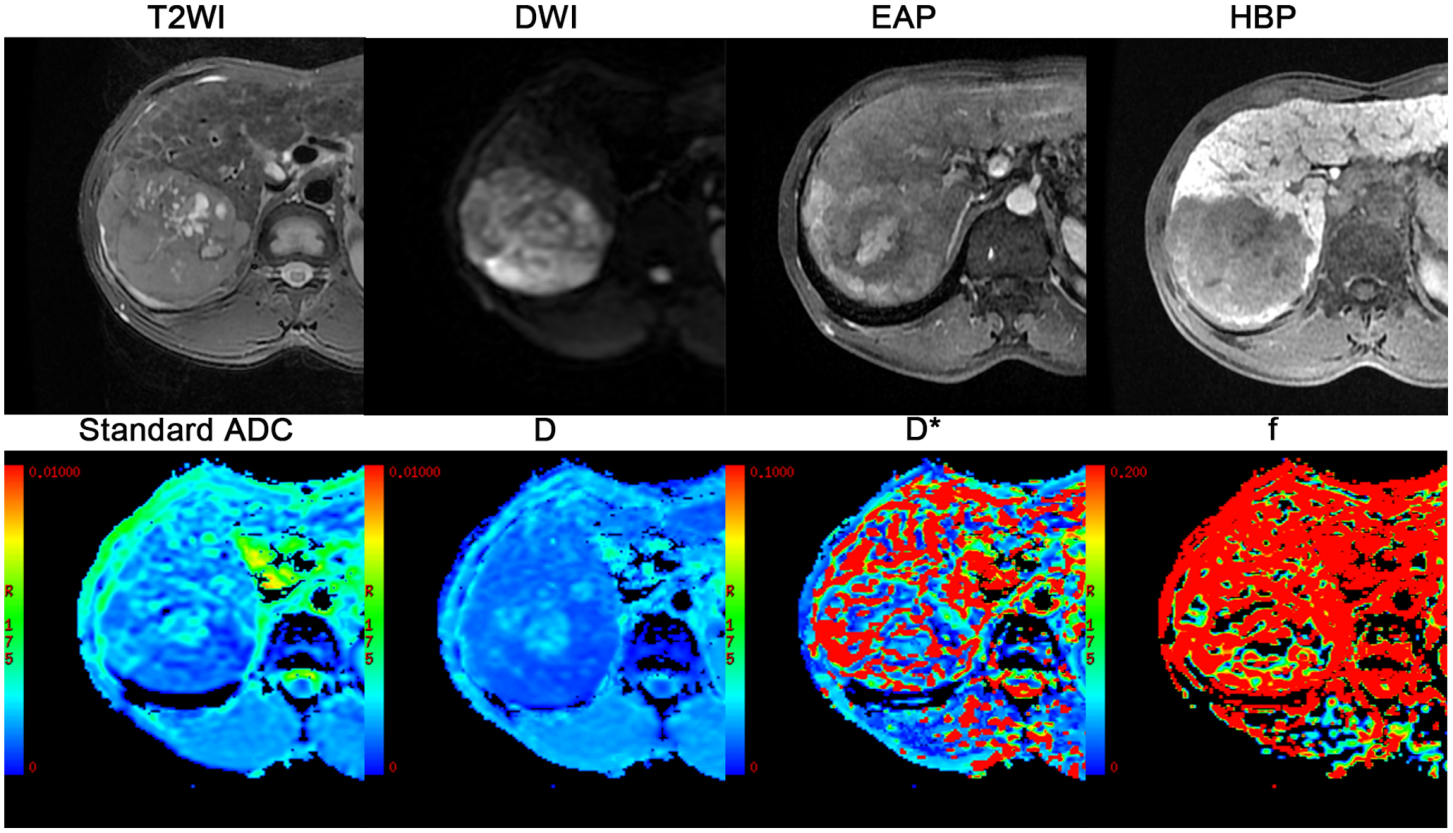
A 45-year-old man with chronic hepatitis B and who underwent liver resection for HCC (poorly differentiation) with microvascular invasion. MRI shows a 9.0 cm hyperintensity on axial respiratory-triggered, T2-weighted, fast-spin-echo (FSE) image (T2WI) in right liver with hyperintensity on DW imaging and early arterial-phase(EAP) and a definite hypointensity on hepatobiliary-phase(HBP) images (d), presented with an irregular shape. Corresponding IVIM-derived four parameters (Standard ADC, D, D* *f*) maps, the value of Standard ADC, D, D* f for the lesion was 1.09 × 10^−3^ mm^2^/sec, 0.89× 10^−3^ mm^2^/sec, 24.3× 10^−3^ mm^2^/sec,12.8%, respectively.
